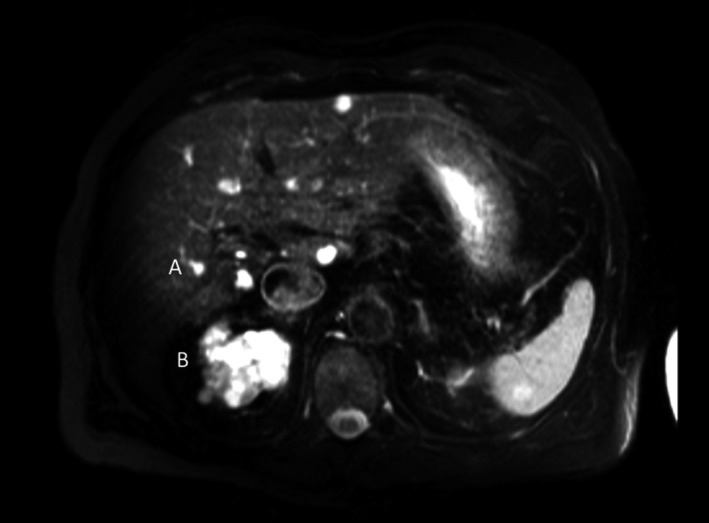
Two years before the development of HCC, T2‐weighted MRI revealed the presence of liver cysts (A) and renal polycystic kidneys.
